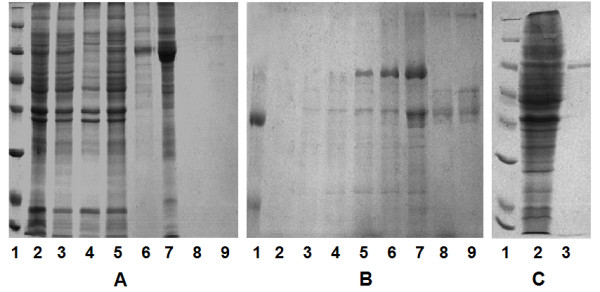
The purification of recombinant multi-mimotope of influenza A virus. a: The multi-mimotope was expressed in soluble form in bacteria and purified with affinity chromatography (lane 1: protein marker: 116.0, 66.2, 45.0, 35.0, 25.0, 18.4, 14.4KDa; lane 2: multi-mimotope gene was transferred to bacteria and induced with IPTG; lane 3: supernatant of ultrasound-broken bacteria; lane 4: pellete of ultrasound-broken bacteria; lane 5: flow-through of supernatant loaded on Ni^2+^-NTA-resin; lane 6: first 0.25 ml elution from 0.25 ml Ni^2+^-NTA-resin with 60 mM imidazole; lane 7: second 0.25 ml elution from Ni^2+^-NTA-resin with 60 mM imidazole; lane 8-9: elution from Ni^2+^-NTA-resin with 1 M imidazole). b: The optimized concentration gradient between 5 and 60 mM imidazole for affinity chromatography (lane 1: protein marker: 97.4, 66.4, 43.0KDa; lane 2-3: elution from Ni^2+^-NTA-resin with 5 mM imidazole; lane 4-5: elution from Ni^2+^-NTA-resin with 10 mM imidazole; lane 6-7: elution from Ni^2+^-NTA-resin with 30 mM imidazole; lane 8-9: elution from Ni^2+^-NTA-resin with 60 mM imidazole). c: The multi-mimotope was purified with repeat affinity chromatography (lane 1: protein marker: 116.0, 66.2, 45.0, 35.0, 25.0, 18.4, 14.4KDa; lane 2: supernatant of ultrasound-broken bacteria; lane 3: multi-mimotope purified by repeat affinity chromatography).
